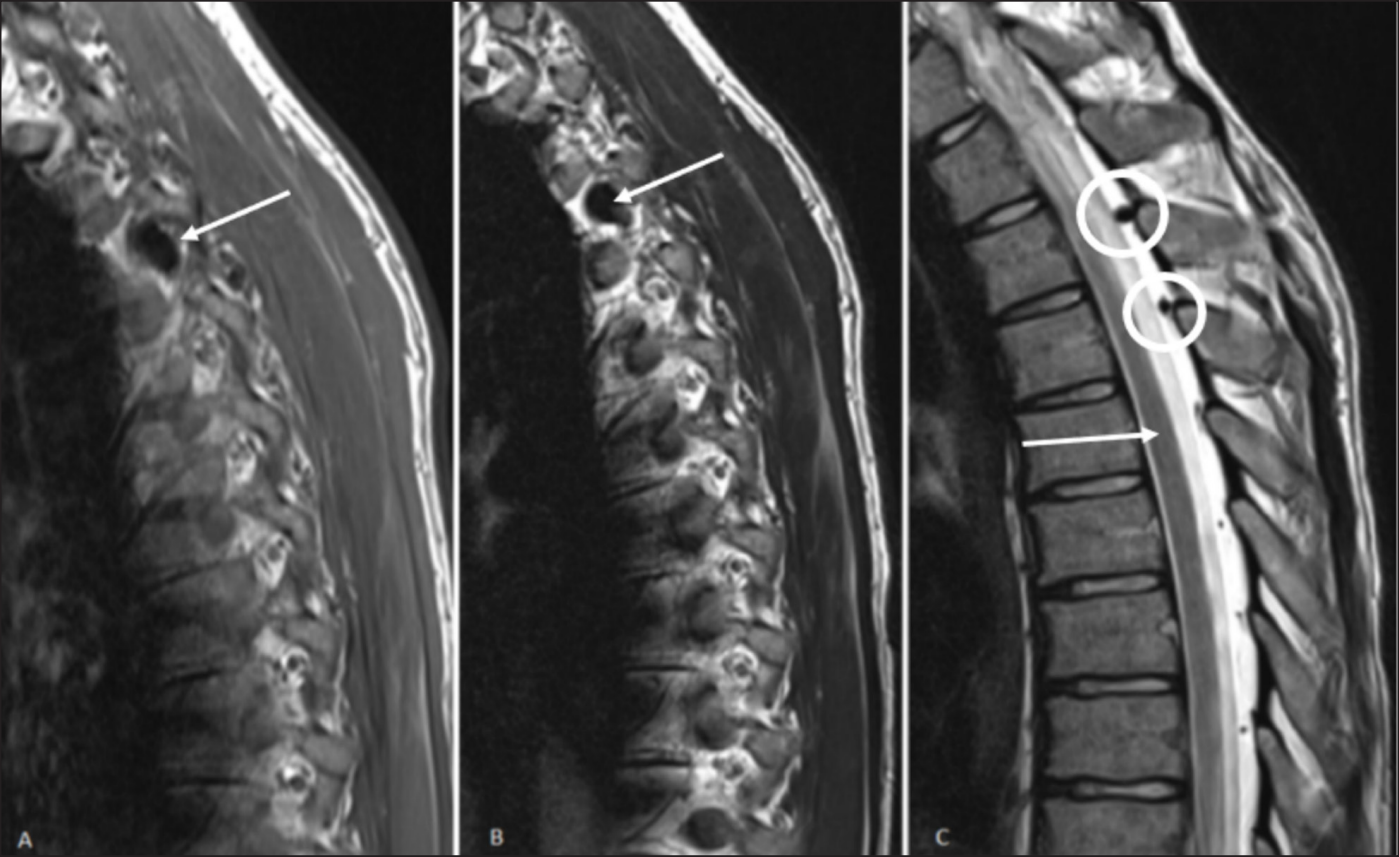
To better delineate these vascular structures and to exclude associated intrathoracic abnormalities, an additional computed tomography (CT) imaging was performed with injection of intravenous contrast material (both venous and arterial phase were acquired). This demonstrated an azygos lobe in the right lung (Figure 2A, semi-axial MIP, arrows). Visualized azygos vein passed further through the right Th3-Th4 neural foramen (Figure 2B, semi-axial MIP, Figure 2C, semi-coronal reformation, arrows and Figure 3, 3D reformatting) and ran caudally intraspinal in the right extradural space to level Th12 (Figure 2C, arrow heads). No additional intrathoracic vascular abnormalities were detected.
